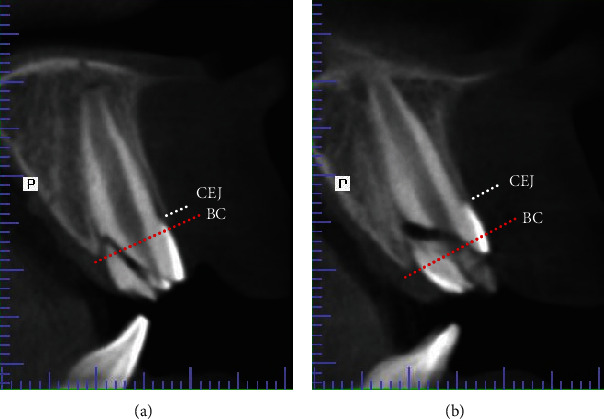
Sagittal CBCT views of (a) #11 and (b) #21 showing multiple fracture lines and loose fragments. From the image scale, the fracture lines extend subcrestally 2 mm in #11 and 5 mm in #21. The dotted lines represent CEJ and bone crest (BC). Therefore, the level of intrusion is approximately 3 mm in #11 and 6 mm in # 21. Root development shows immature apical formation (open apex) in both teeth.
